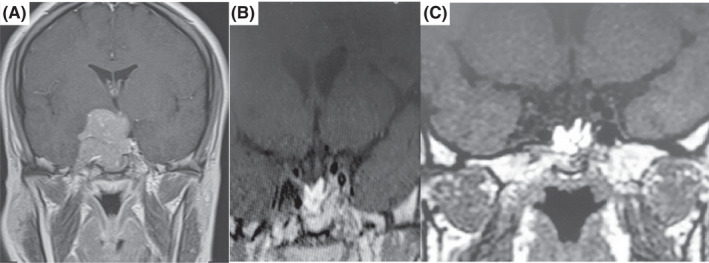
(A) Coronal image of pituitary gland MRI post‐contrast shows large mass obliterating suprasellar cistern with extension into the third ventricular floor, right middle cranial fossa, and right cavernous sinus extension. (B) Post second surgery and radiation study. (C) One‐year post‐TMZ treatment shows a significant decrease in tumor size with a small residual lesion
